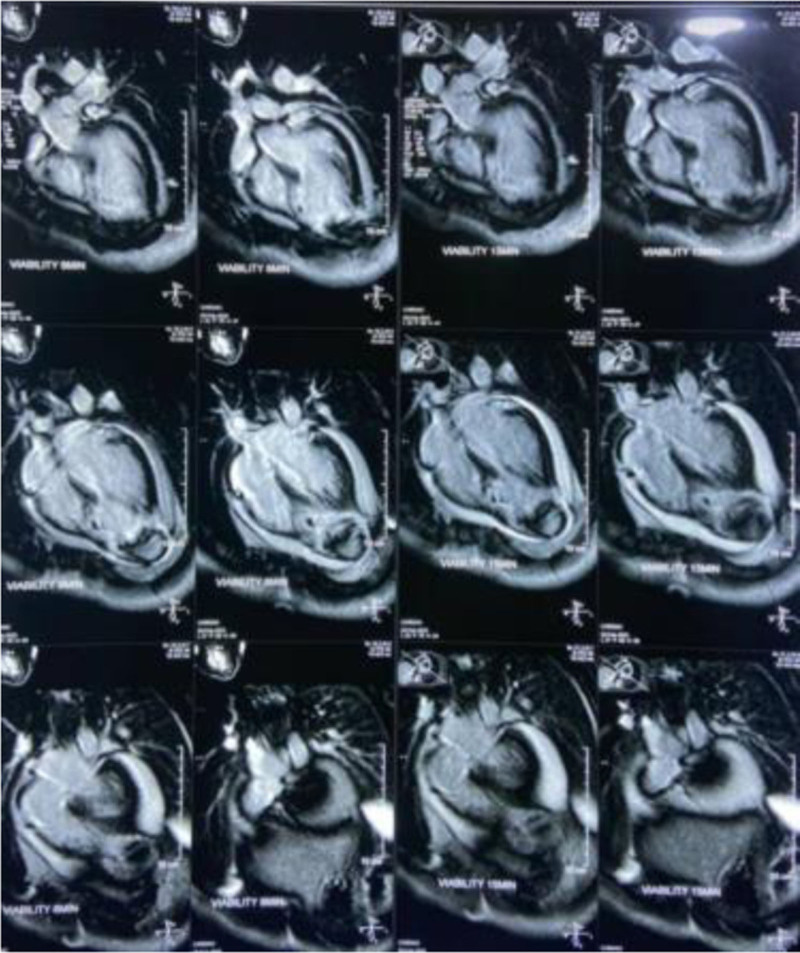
The cardiac MRI series showing wall thinning in the anterolateral region. And hypointense areas indicative of mural thrombus formation. MRI = magnetic resonance imaging.
